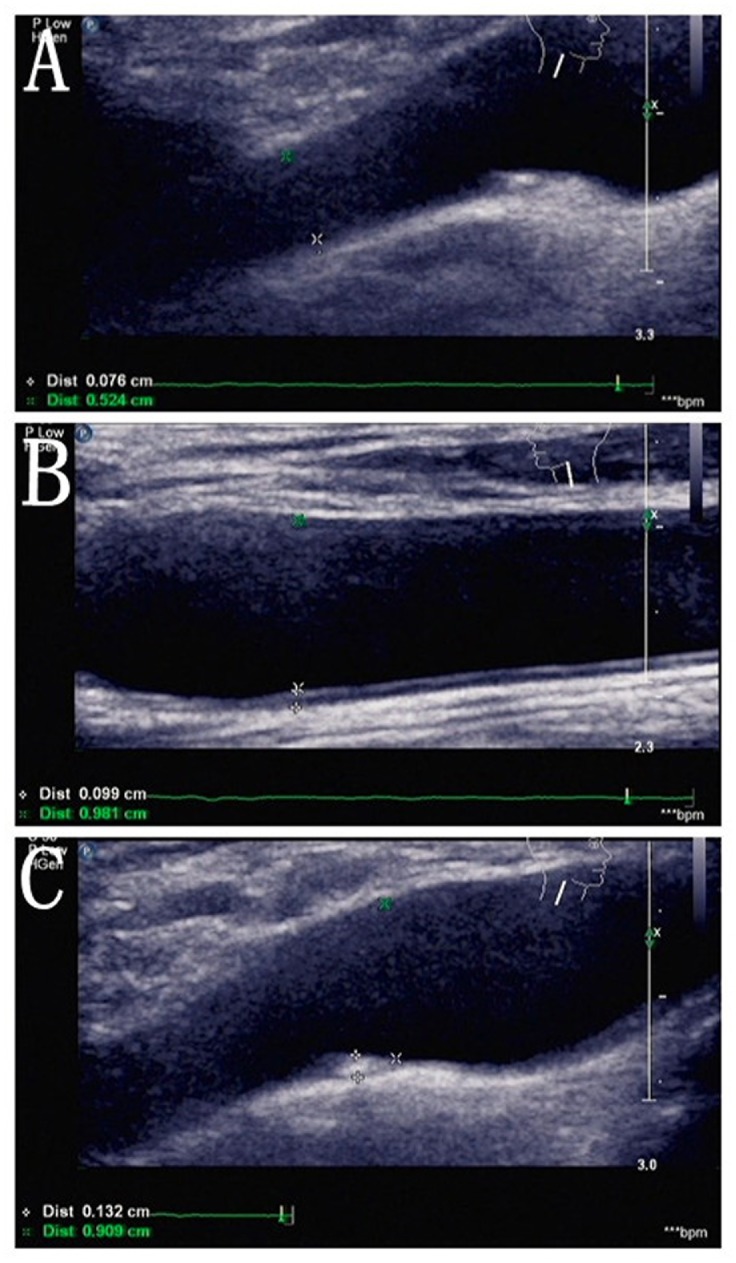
Carotid ultrasound image. *Representing thickening or plaque of carotid artery. (A) Normal carotid intima; (B) Carotid intimal thickening; (C) Carotid artery plaque.
